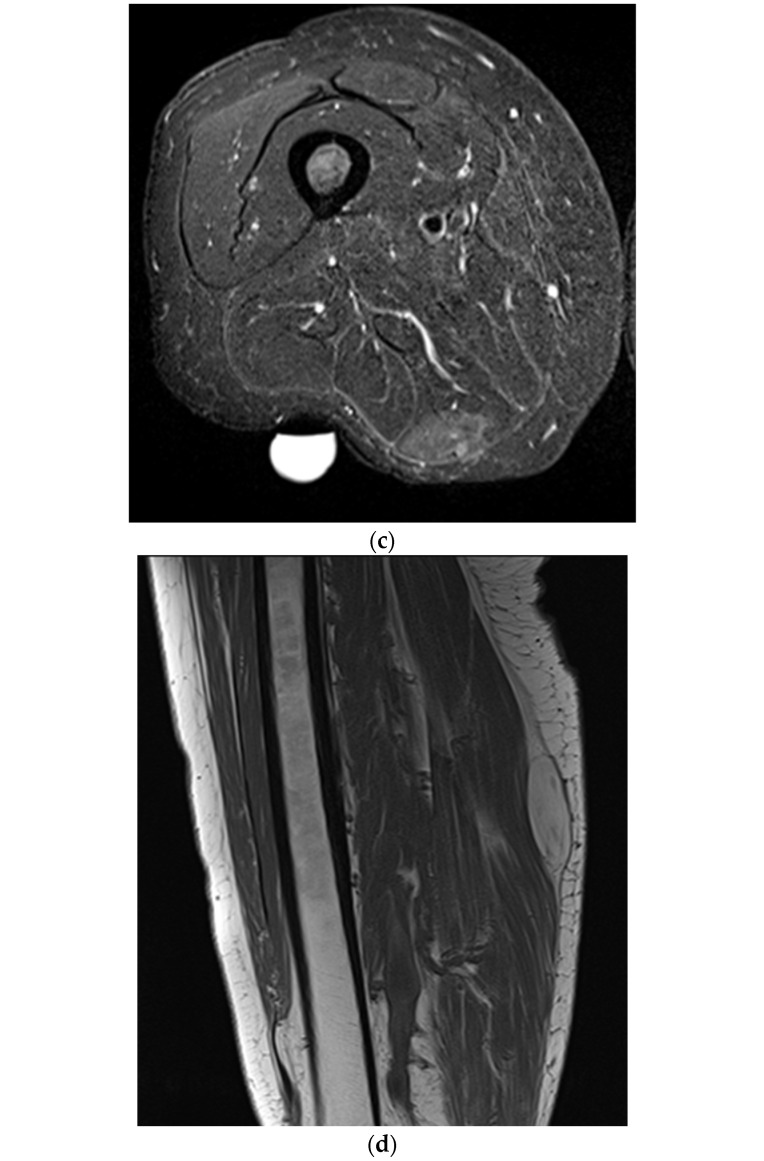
A 79-year-old woman operated on for a high-grade liposarcoma. At follow-up, both US (a) and MRI (b–d) detected a solid nodule in the posterior aspect of the thigh with prevalent fatty content near the surgical area. Both US and MRI were reported as consistent with recurrence; however, pathology did not show any malignant tissue after surgery.
